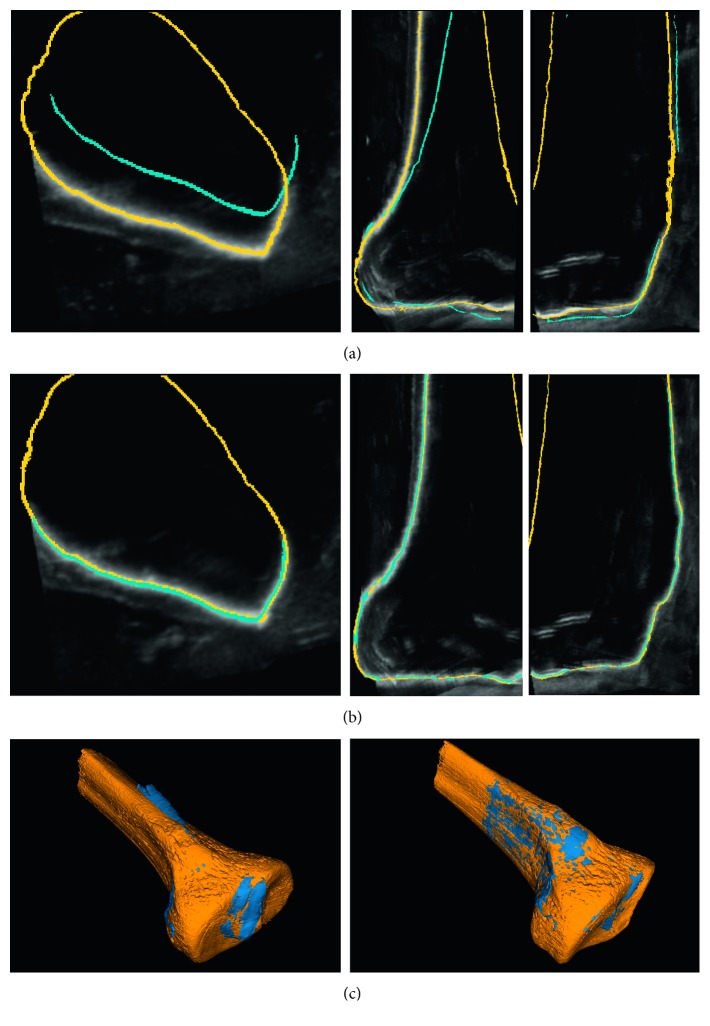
(a) Orthogonal views of the ultrasound volume with the unregistered ultrasound mesh (shown in blue) and registered reference CT mesh (shown in yellow). (b) Orthogonal views of the ultrasound volume with the registered ultrasound and CT meshes overlapped. (c) Ultrasound (blue) and CT (yellow) 3D meshes before (left) and after (right) registration.
